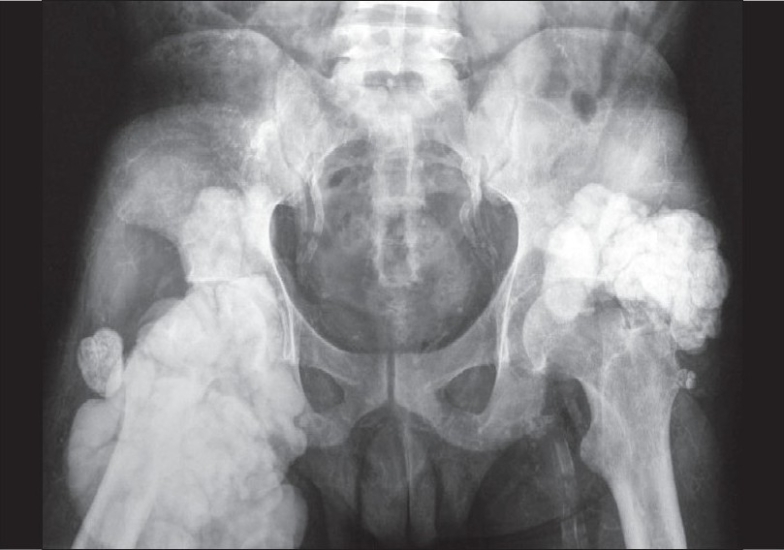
X-ray of the pelvis with both hips
